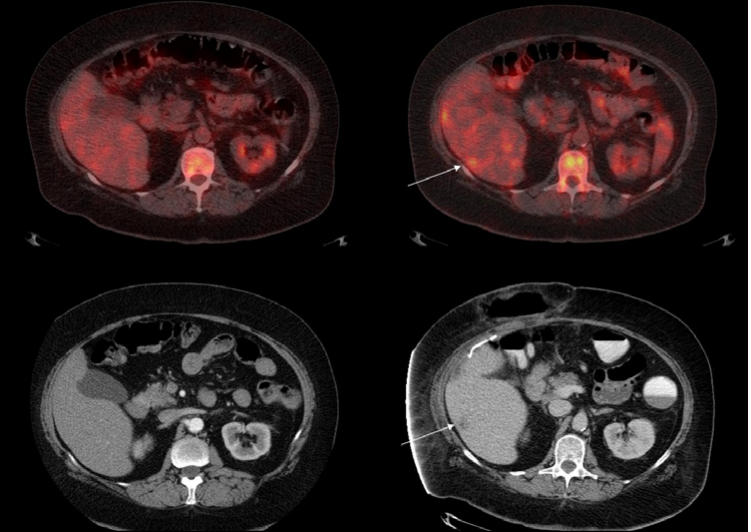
Early (top left) and delayed phase (top right) FDG-PET/CT of a 54 year old female with metastatic colorectal carcinoma showing a segment 6 lesion not seen on CECT performed on the same date (bottom left) but surfacing 6 months later (bottom right).
